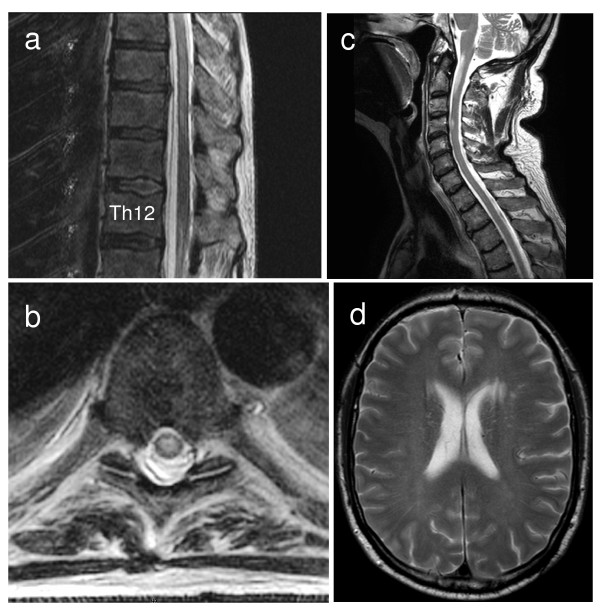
MRI data of patient 1 (a, b) and patient 2 (c, d). Saggital (a) and axial (b) T2-weighted magnetic resonance images show increased signal intensity in the spinal cord of patient 1. The lesion extends from the T5 to the T10 vertebral body. The axial image shows the spinal cord at the level of the T6 vertebral body. (c) T2-hyperintense lesion on the level of the C4 vertebral body of patient 2. (d) Brain-MRI shows a single T2-hyperintense paraventricular left hemispheric lesion in patient 2.
